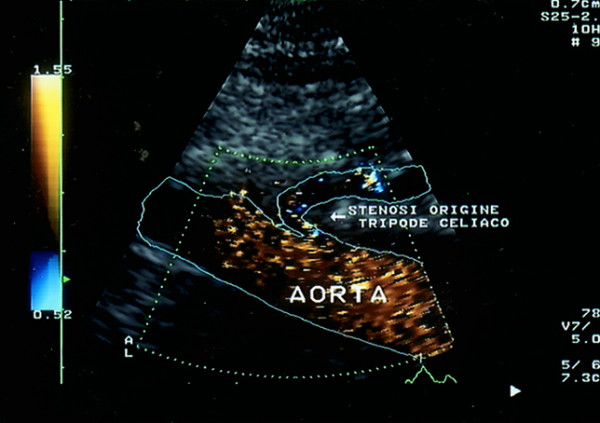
Color Doppler of aorta : celiac artery stenosis (longitudinal proiection)
